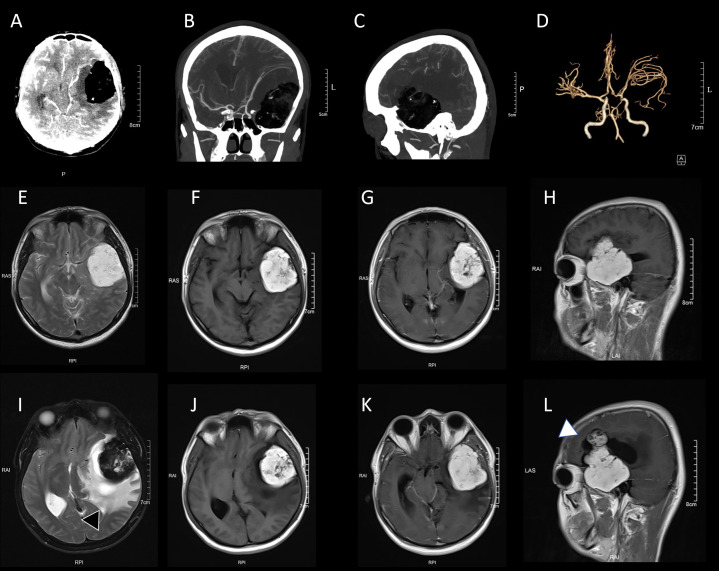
Imaging findings of the case. (A–D) Baseline computed tomography angiography (CTA) of the brain revealing a left frontotemporal mass with superior displacement and compression of the left common carotid artery. Images include axial view (A), maximum intensity projection (MIP) coronal view (B), MIP sagittal view (C), and 3D vascular reconstruction (D). (E–H) Baseline contrast-enhanced brain magnetic resonance imaging (MRI) demonstrating a large left temporal mass with heterogeneous short T1 and long T2 signals, measuring approximately 47 mm × 59 mm × 72 mm. Fat suppression shows low signal intensity, suggesting high fat content. Post-contrast imaging reveals patchy enhancement within the lesion and nodular enhancement at the superior margin, with compression of surrounding tissues. Images include T2-weighted axial view (E), T1-weighted axial view (F), T1-weighted post-contrast axial view (G), and T1-weighted post-contrast sagittal view (H). (I–L) Follow-up contrast-enhanced MRI performed one year later shows persistence of a large left temporal mass with heterogeneous short-T1 and long-T2 signals. Compared with baseline, the enhancement has become more prominent (white arrows), with progression of perilesional edema and mass effect (black arrows). Images include T2-weighted axial (I), T1-weighted axial (J), T1-weighted post-contrast axial (K), and T1-weighted post-contrast sagittal (L) views.
